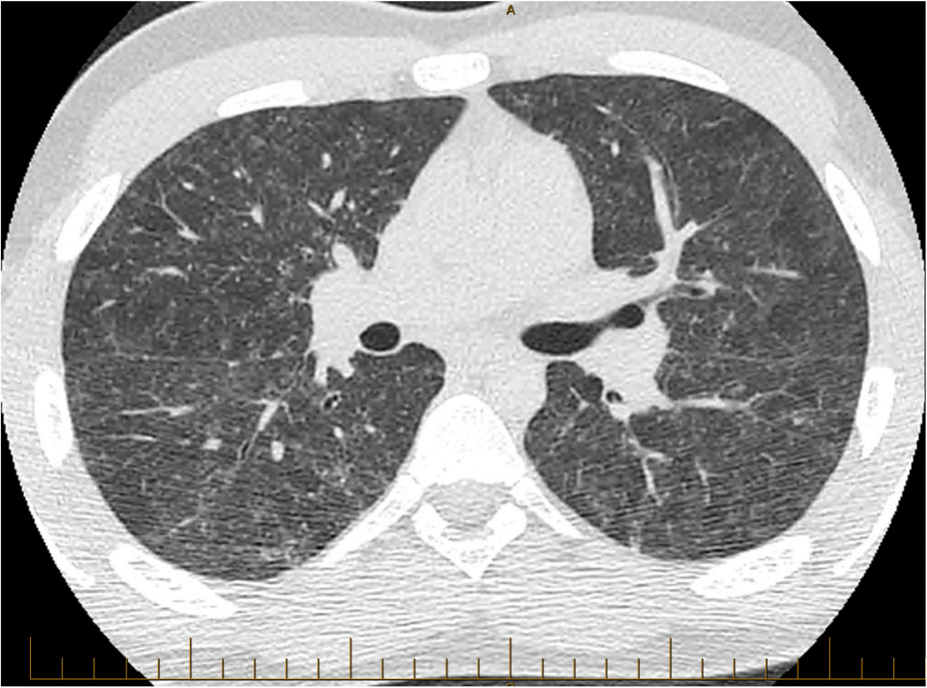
Computed tomography (CT) scan of the patient at diagnosis demonstrating bilateral, symmetrical, diffuse patchy ground‐glass opacities with associated small volume adenopathy, consistent with hypersensitivity pneumonitis (HP)‐associated non‐tuberculous mycobacteria (NTM) infection.
